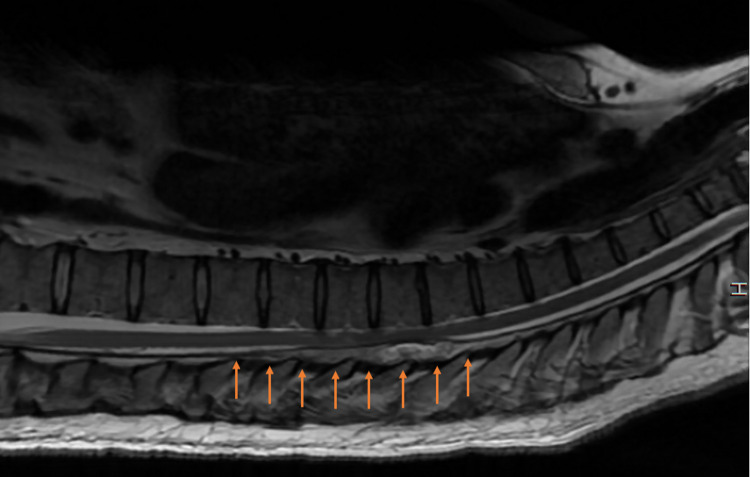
MRI showing abnormal enhancement (arrows) in the dorsal epidural space at T5-T9 levels.
